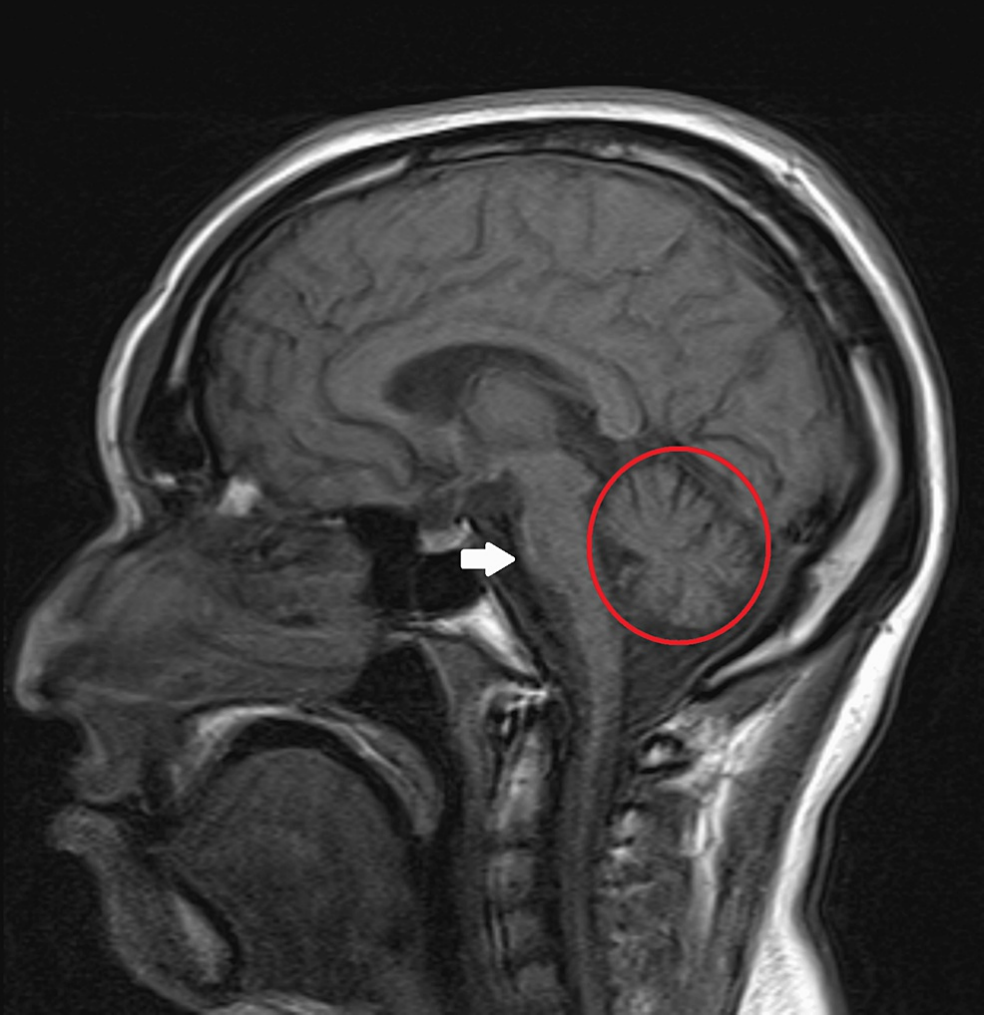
Brain magnetic resonance imaging revealed cerebellar atrophy, with widening of the sulci of the cerebellar hemispheres (red circle) and brain stem atrophy (white arrow).
